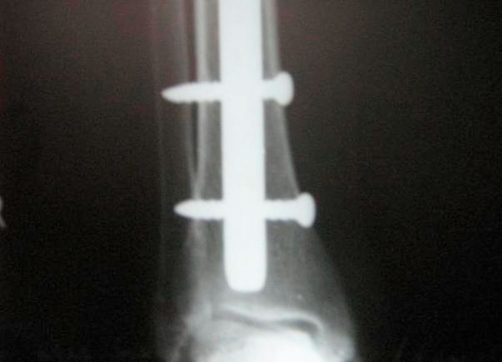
Completed nailing with interlocking bolts in position. Picture showing the distal locking bolts of the nail in place. This radiograph was taken at one and half years after initial procedure.
